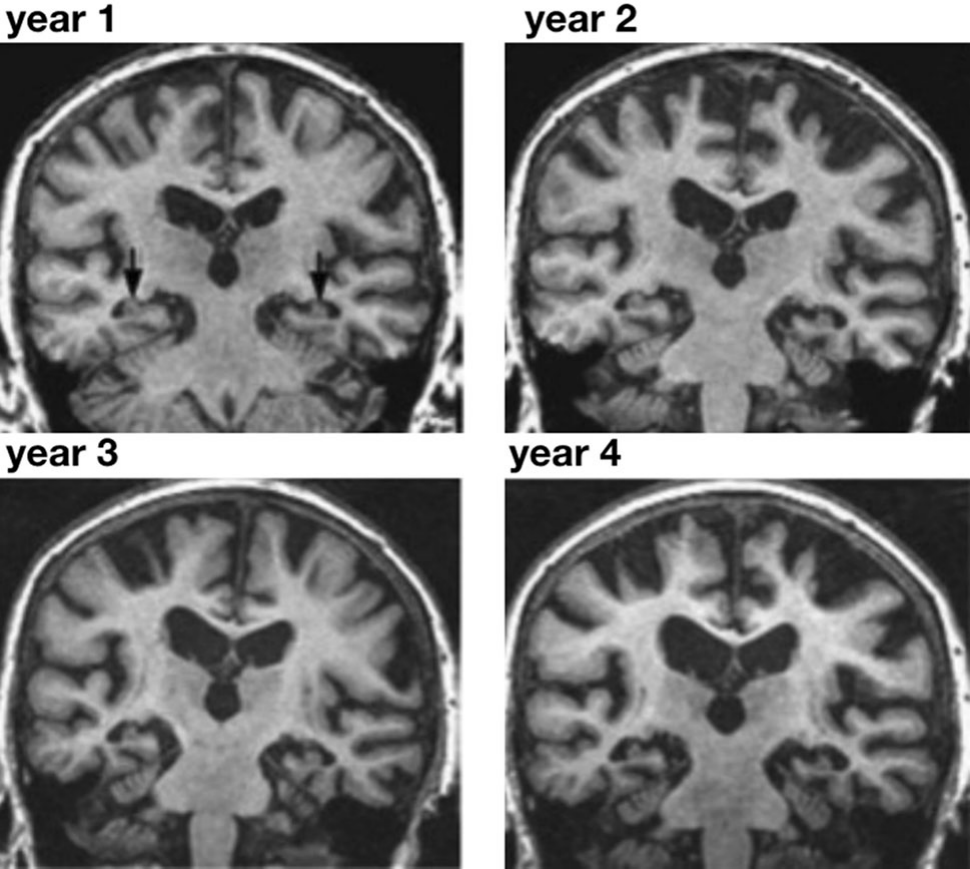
Cerebral atrophy in neurodegeneration. Longitudinal magnetic resonance imaging of an Alzheimer’s patient reveals the characteristic pattern of progressive atrophy in the hippocampus, a widening of the cortical sulci, a gradual enlargement of the ventricles, a pronounced cortical thinning, and a shrinking of the hippocampus. Adopted with permission from Ref. 39.
